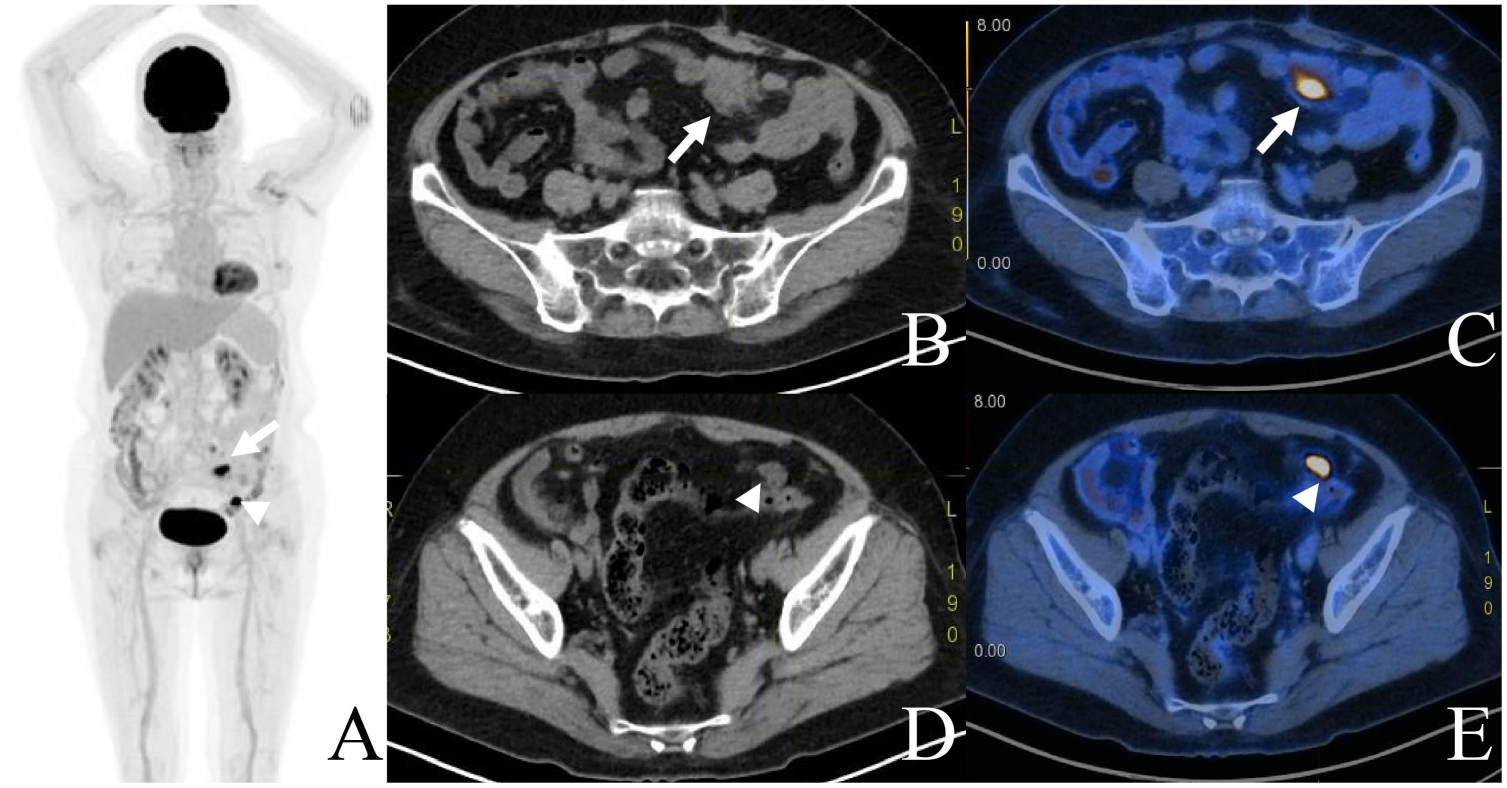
The 18F-FDG PET/CT imaging 11 months after chemotherapy revealed intense uptake in the mesentery (arrows) and peritoneum (arrowheads) around surgical anastomosis, with a SUVmax of 16.3 [(A) MIP, (B, D) CT, and (C, E) fusion].
